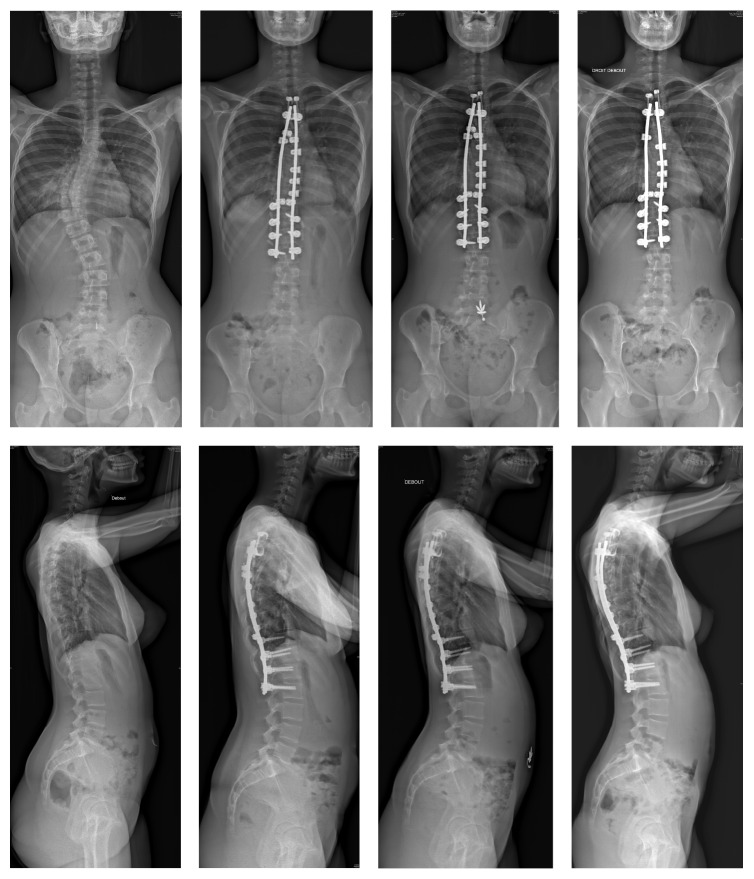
Preoperative, postoperative, and 2-year follow-up radiographs. The patient presented a Lenke 1 scoliosis with severe hypokyphosis. Hybrid instrumentation with sublaminar bands allowed correction of the deformity in frontal plane but also the restoration of the thoracic kyphosis. At last follow-up, the correction in both frontal and sagittal planes is maintained and sagittal alignment is satisfactory.
